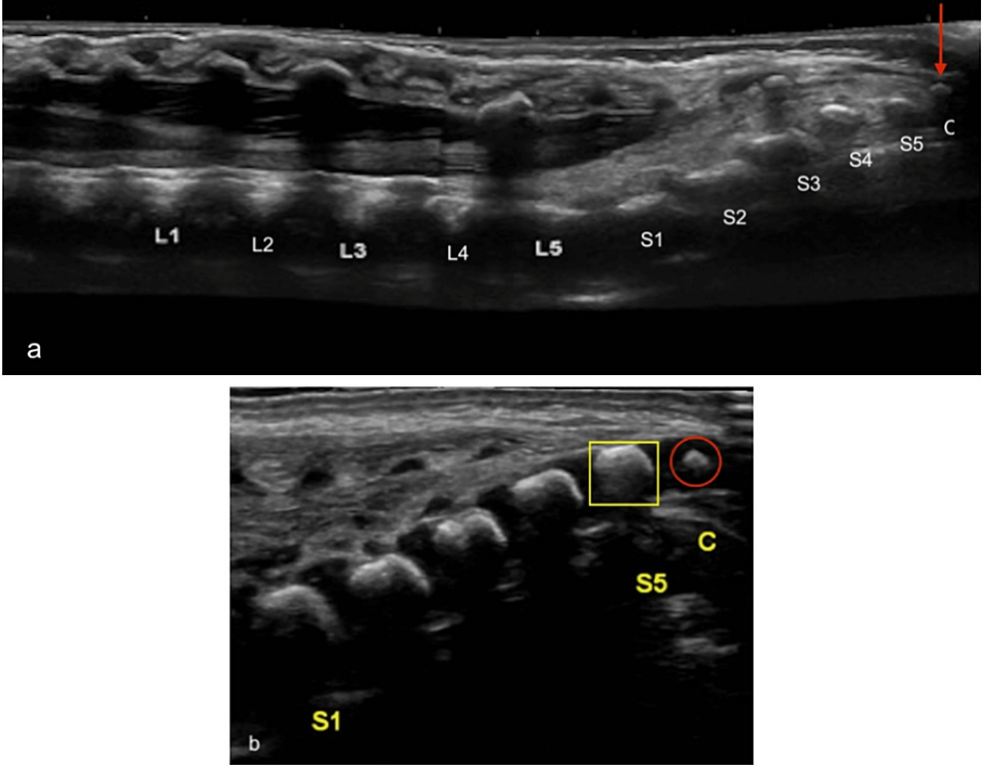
Coccyx appearance and location (a) Longitudinal ultrasound image demonstrating the coccyx (red arrow) as the last echogenic structure at the distal end of the spine. (b) The ossification center of the coccyx has a rounded configuration (red circle), as opposed to the squarish appearance (yellow square) of sacral ossification centers Radiological images obtained by the authors
